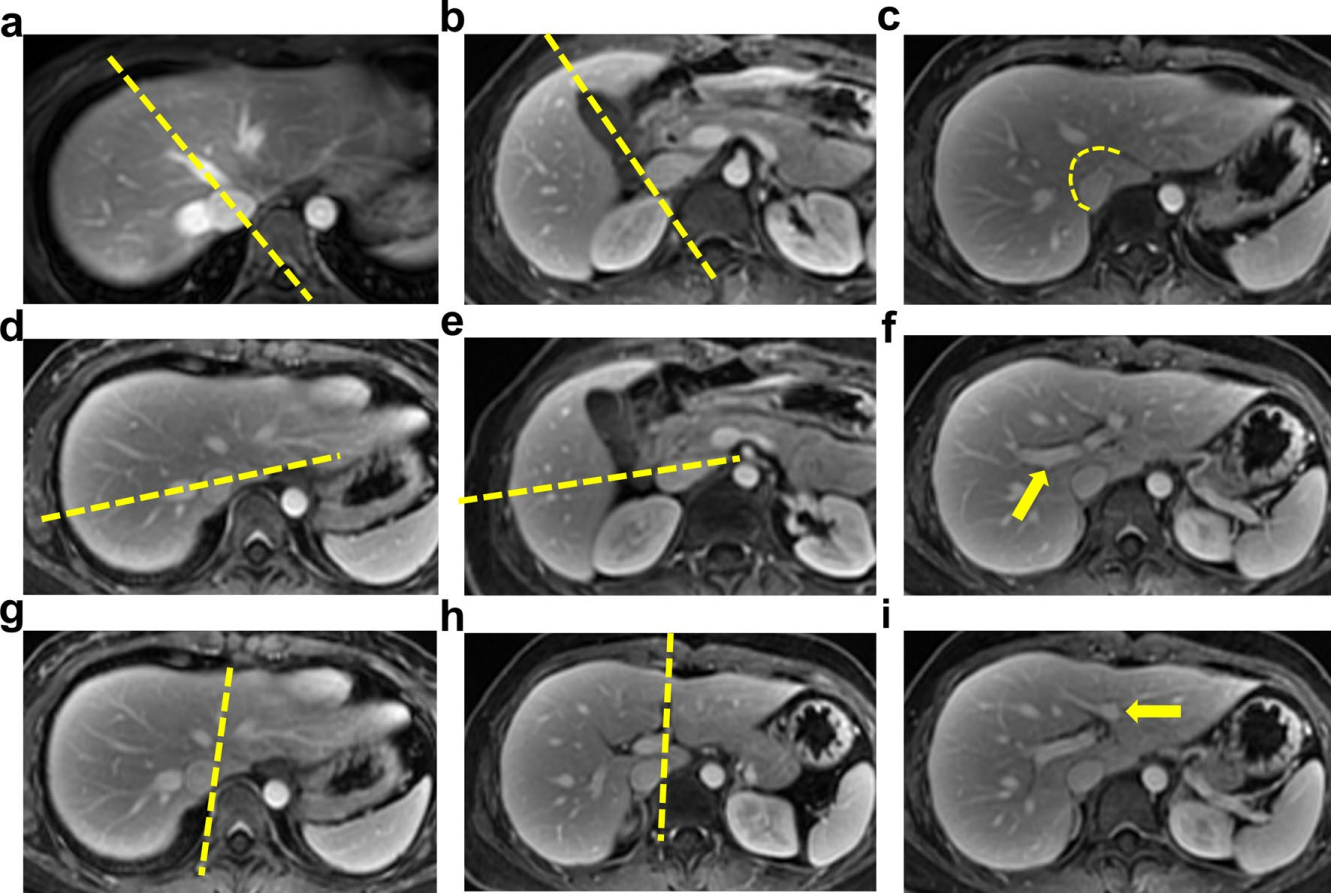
Method of Couinaud’s classification on axial MRI images. Five planes (three vertical planes along the main stem of hepatic veins and two horizontal planes through the primary branches of the portal vein) are used to divide the liver. In axial MR images, the line from the midpoint of the IVC to the MHV or vertex of the gallbladder fossa divides the right and left lobes (a and b). The RHV further divides the right lobe into anterior and posterior segments (d and e). The LHV (in the upper part of the liver) and the left longitudinal fissure (in the lower part of the liver) divide the left lobe into a medial and a lateral segment. (g and h). Each segment of right and left lateral lobes is further divided into a superior segment and an inferior segment by the main stem of the right portal vein and the sagittal part of the left portal vein (f and i). The caudate lobe is separated from the other segments by the natural curve from the venous ligament fissure to the right wall of the IVC (c). IVC, inferior vena cava; MHV, middle hepatic vein; RHV, right hepatic vein; LHV, left hepatic vein.
